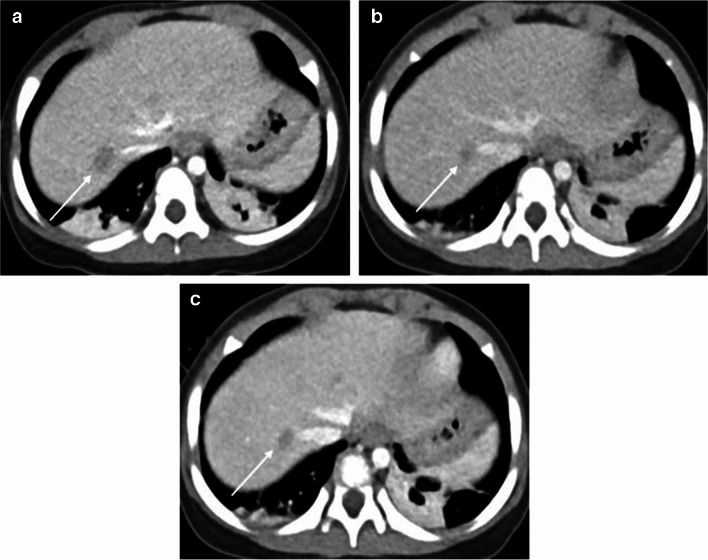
Axial contrast-enhanced computed tomography (CT) images of a 1-year-old girl with adrenal gland neuroblastoma and liver metastasis. Sequential abdominal CT was conducted within 3 months. a Standard-dose image shows a 1.3 cm relatively well-defined low attenuated metastatic nodule in liver segment 7/8 (*arrow*). b Low-dose image shows a 1.1-cm ill-defined low attenuated metastatic nodule in liver segment 7/8 (*arrow*). c In the contrast-boosted image, the nodule looks more well-defined (*arrow*) and the lesion conspicuity is improved compared to the low-dose image. The qualitative lesion conspicuity scores of the standard-dose, low-dose, and contrast-boosted images were 4/4, 3/3, and 4/4, respectively (reader 1’s score/reader 2’s score)
